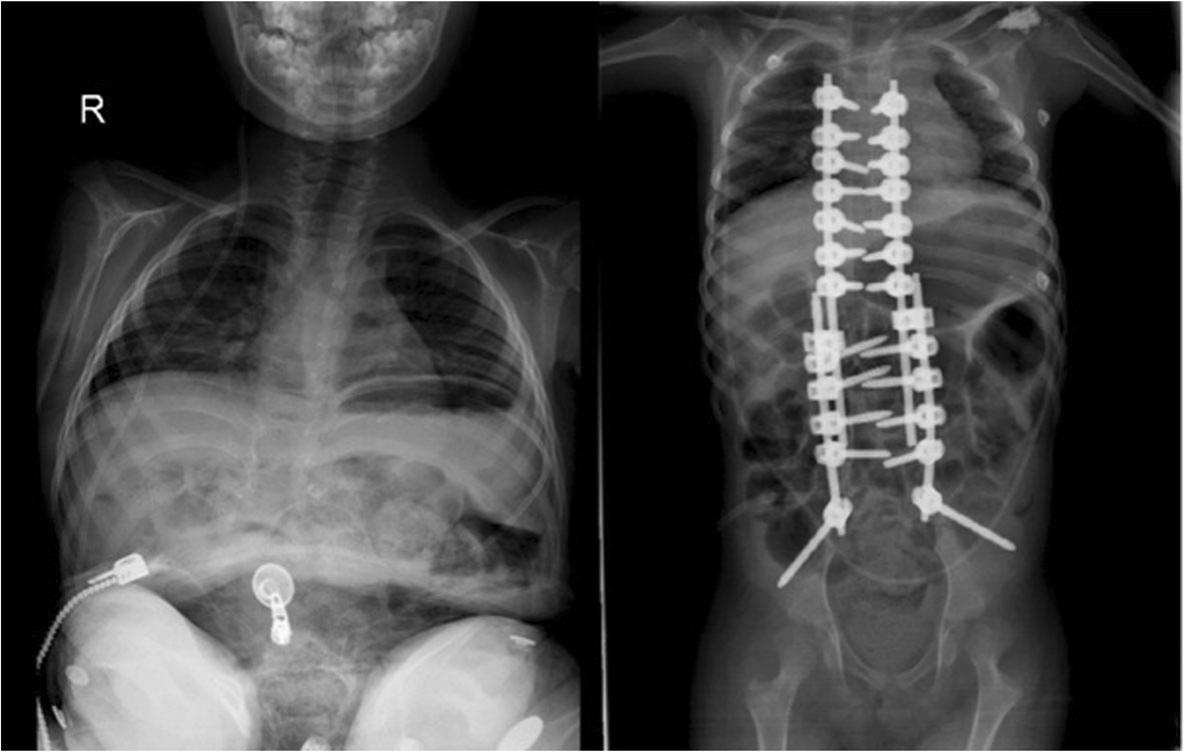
Pre- and early postoperative anteroposterior (AP) radiography
